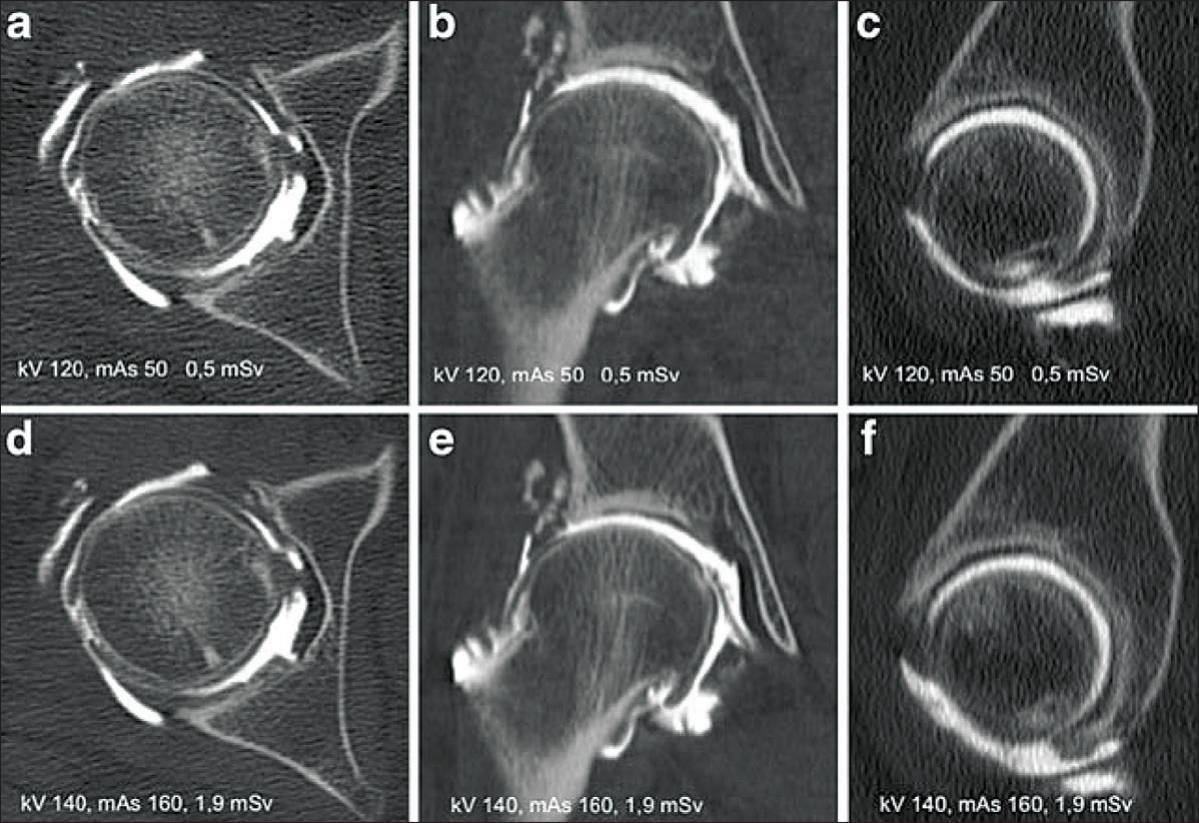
CTA of the hip joint on a patient with a body mass index of 28 kg/m^2^ and acquisition using 120 kVp and 50 mAs. (a) Axial, (b) Coronal, (c) Sagittal multiplanar reconstruction (MPR) and axial (d) and Coronal (e) and Sagittal (f) Reformats obtained with the routine dose of 140 kVp and 160 mAs. Note that even if the noise is higher in the images obtained with the lowest dose (a, b, c), the depiction of the contrast-cartilage and cartilage-subchondral bone plate interfaces is optimal compared with the high-dose standard protocol (d, e, f). Cartilage defect in the upper pole of the femoral head, visible in the coronal reformatted images (b, e), is well depicted by the low-dose protocol (b) without substantial difference from the high-dose protocol (e).
